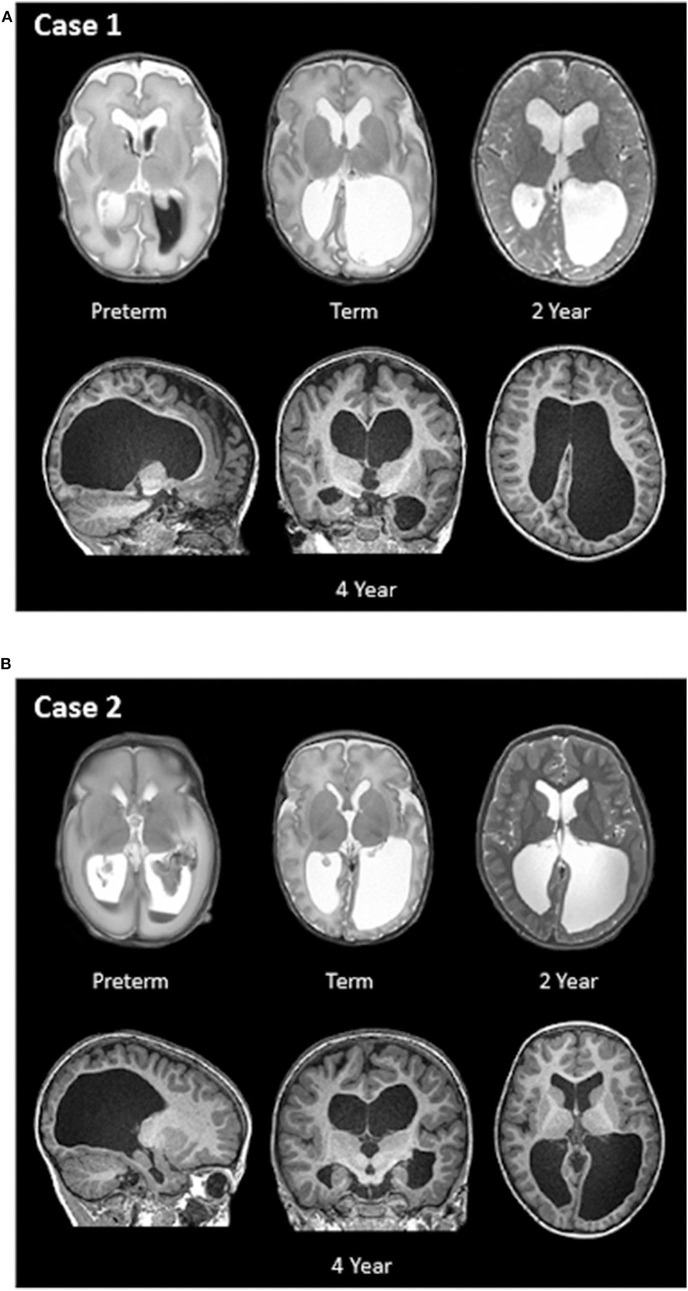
(A,B) Longitudinal structural MR images of Case 1 and Case 2. T2- and T1-weighted MRI images were obtained at four different ages: within two weeks of birth (preterm; top left), term-equivalent age (term; top middle), 2 years of age (top right), and 4 years of age (bottom row).
